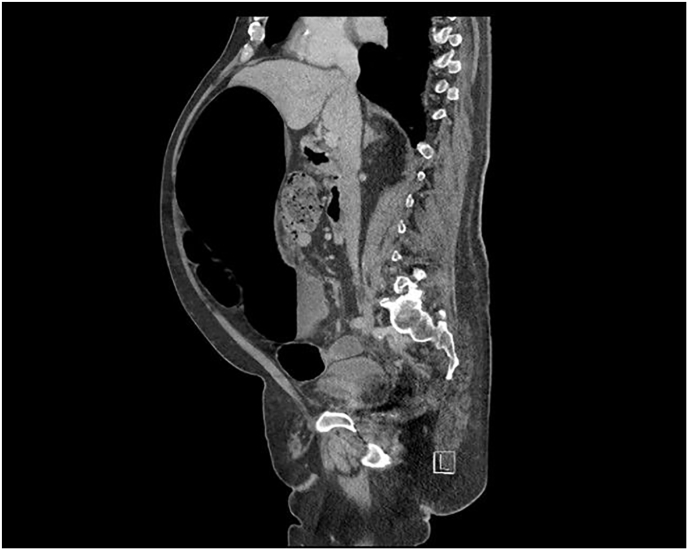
Sigmoid Volvulus Sagittal CT abdomen/pelvis.
